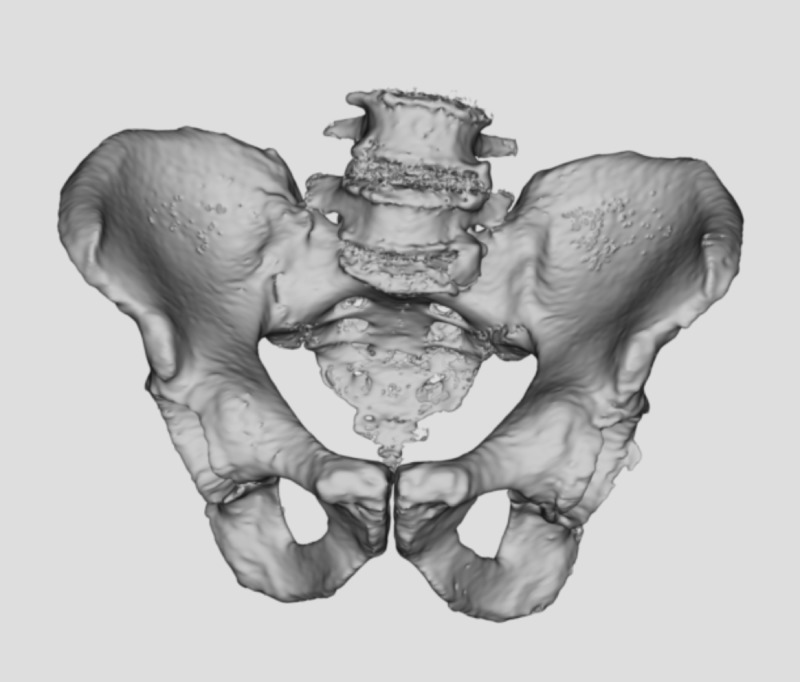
3D rendering created from CT scan
